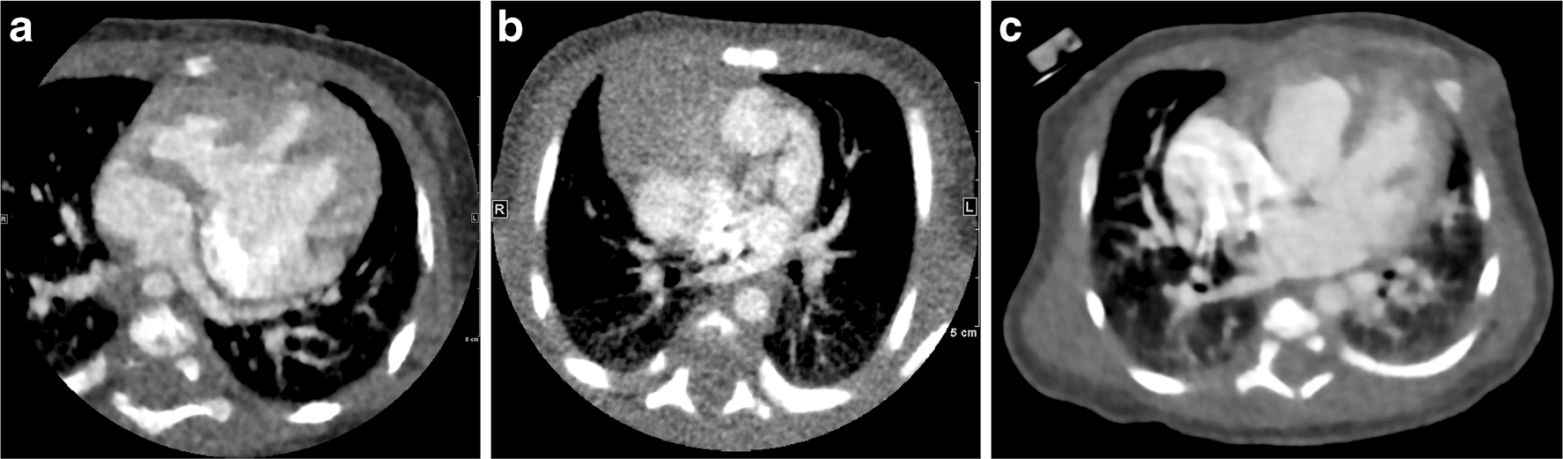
Representative axial contrast-enhanced CT images for the three levels of beam-hardening artifacts. a Score 3, no beam-hardening artifact, in a 1-month-old boy with single atrium, single ventricle, triscupid atresia and double-outlet of the right ventricle. b Score 2, mild artifact, defined as images that still allow evaluation of the surrounding anatomical structures. This image is in a 7-day-old boy with single atrium, single ventricle, pulmonary artery stenosis and total anomalous pulmonary venous return. c Score 1, severe artifacts that preclude evaluation, in a 10-day-old girl with atrial septal defects and ventricular septal defect
